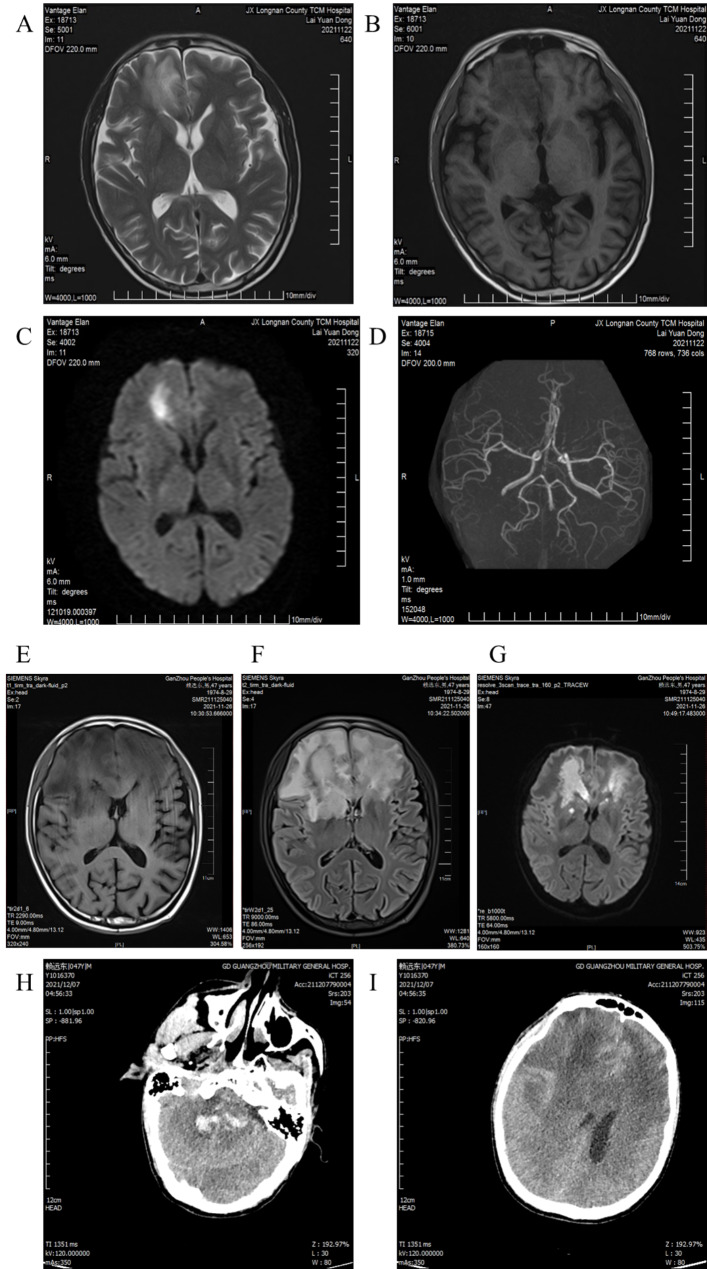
Cranial MRI and MRA images of the patient. A-D, Images acquired one week before admission. E-G, Images acquired on the second day of admission. H-I, Images acquired on the eighth day of admission
